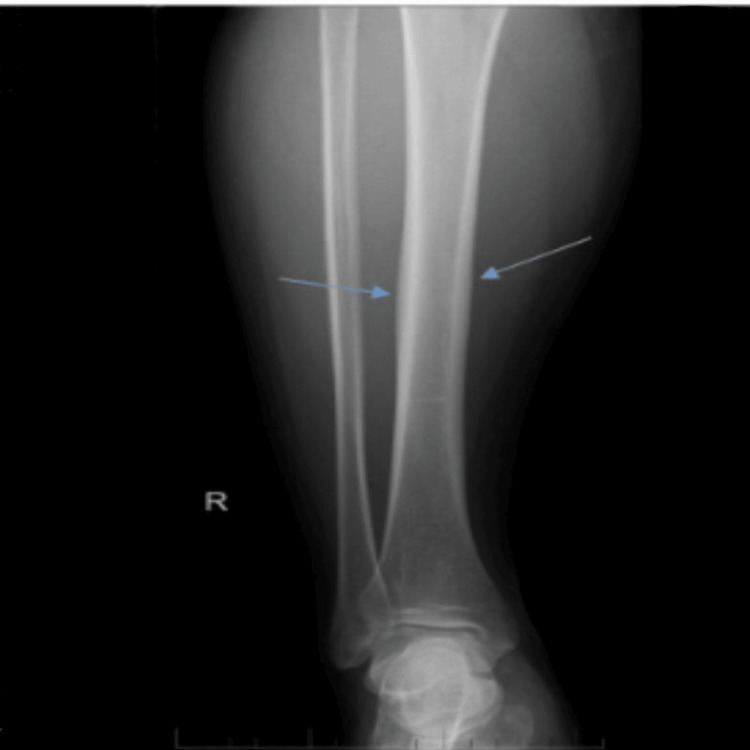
Anteroposterior (AP) radiograph of the right leg showing cortical widening and thickening of the diaphysis. The blue arrows indicate the affected areas.
